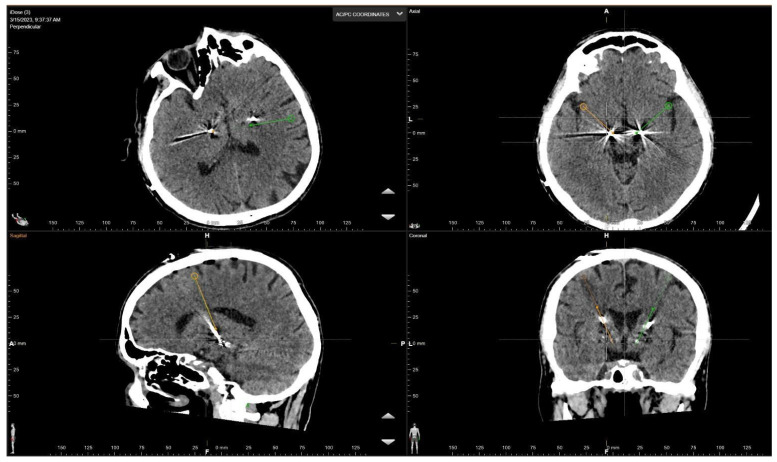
Postoperative CT scan showing the final position of the electrodes obtained after microelectrode recordings.
